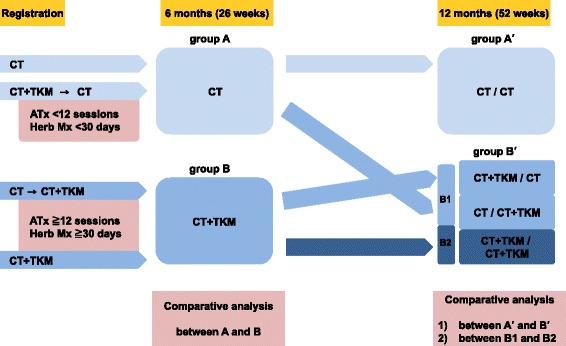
Process and time line of the observational study. CT, conventional treatment; TKM, traditional Korean medicine; ATx, acupuncture; Mx, medication
